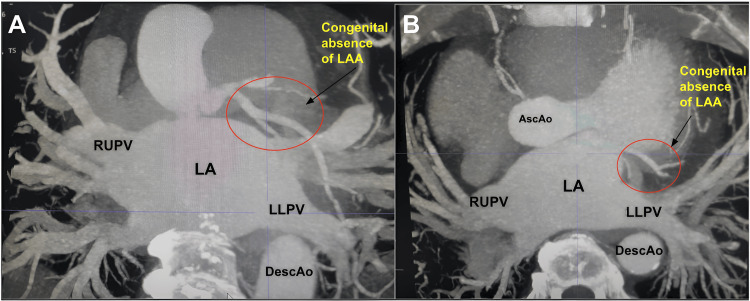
Confirmational cardiac CT scan RUPV = Right upper pulmonary vein, LA = Left atrium, LLPV = Left lower pulmonary vein, DescAo = Descending aorta, AscAo = Ascending aorta, LAA = Left atrial appendage (A) The red circle showing congenital absence of LAA. The cardiac CT scan was done to confirm the results of the TEE, and it shows no continuity of the left atrial appendage with the left atrium supporting the diagnosis of congenital absence of left atrial appendage. (B) Secondary angle view supporting the congenital absence of LAA outlined by the red circle.
